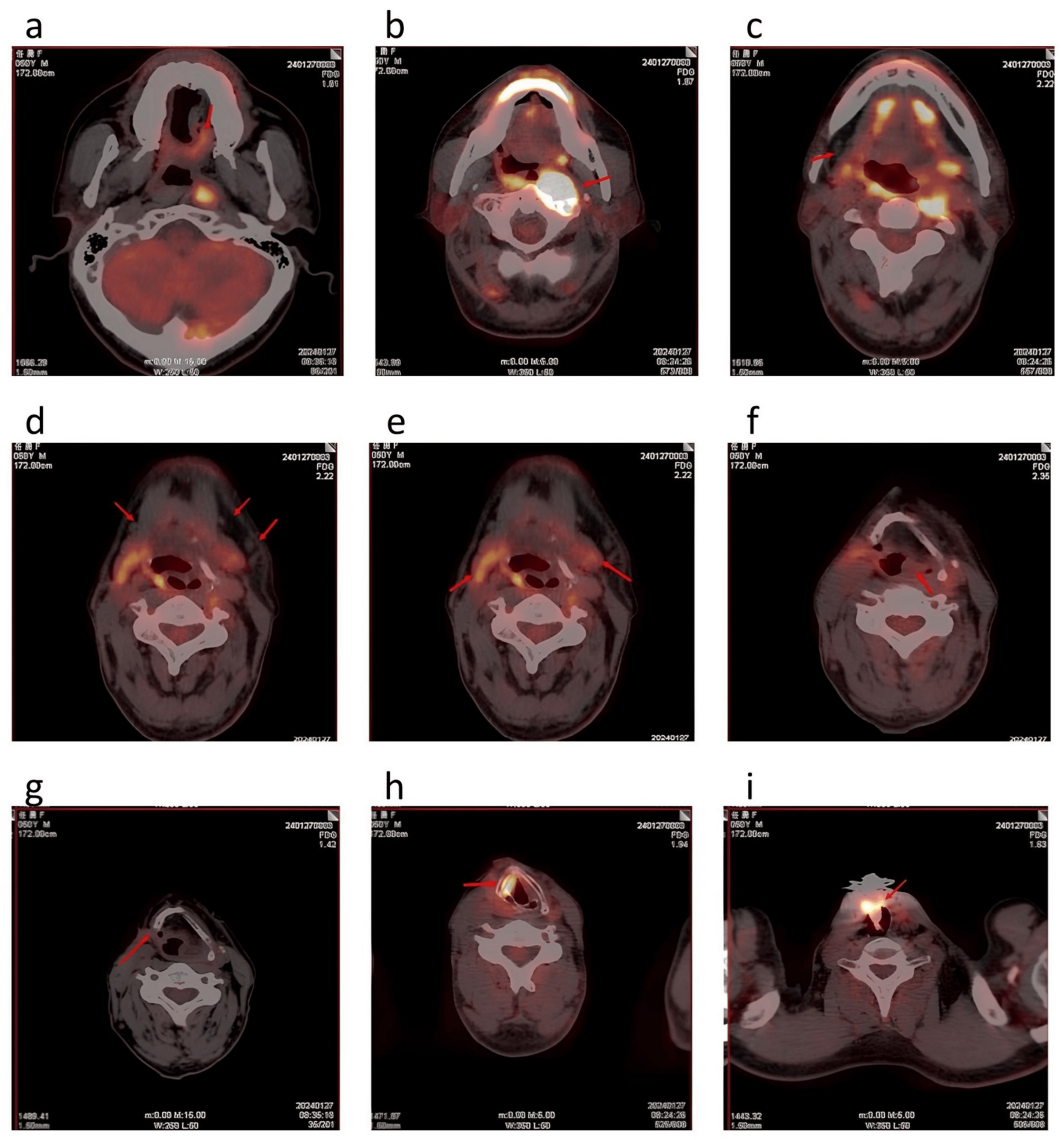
The patient's PET-CT scan images. 1a: After peripheral insertion of a central venous catheter (PICC), asymmetry of the bilateral pyriform sinuses was observed (red arrow). 1b: A soft tissue density lesion was visible in the left pyriform sinus region, with slightly increased radiotracer uptake (red arrow). 1c: No abnormal lymph nodes were observed in the right neck (red arrow). 1d: Multiple slightly enlarged lymph nodes were detected in bilateral level IB regions, with the largest having a short-axis diameter of approximately 6 mm and slightly increased radiotracer uptake (red arrows). 1e: A soft tissue mass was detected in the left parapharyngeal space and anterior to the cervical vertebra (red arrows), measuring 20 mm × 12 mm × 27 mm (transverse × anteroposterior × craniocaudal). 1f: Increased radiotracer uptake was noted in the muscle tissue surrounding the anterior aspect of the left atlas (C1 vertebra) (red arrow). 1g: Postoperative changes in the trachea were observed. Increased radiotracer uptake was seen in the soft tissue surrounding the anterior trachea (red arrow). 1h: Increased radiotracer uptake was found in the right laryngeal region (red arrow). 1i: Increased radiotracer uptake was detected in the soft palate (red arrow).
